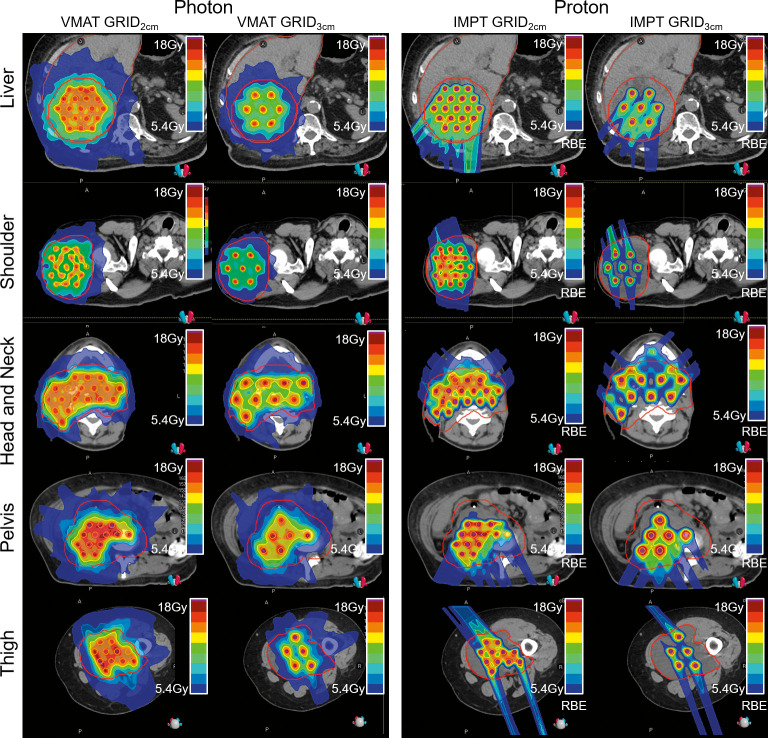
Representative VMAT (left) and IMPT (right) plans are shown with GRID targets at 2- and 3-cm spacing for all 5 planning cases. The GTV is shown in red. Abbreviations: CT, computed tomography; GRID, spatially fractionated radiation therapy; GTV, gross tumor volume; IMPT, intensity modulated proton therapy; VMAT, volumetric modulated arc therapy.
